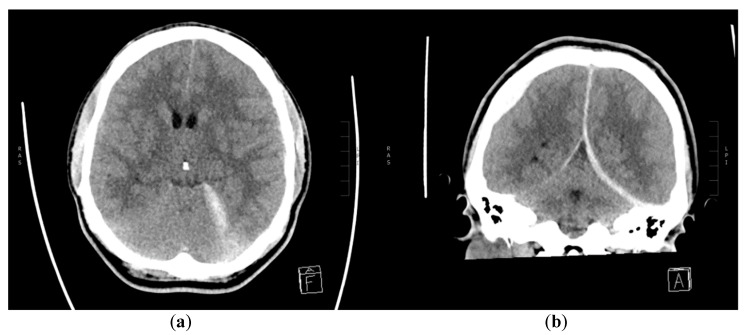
Non-contrast CT scanning of the brain on the day of first admission. (a) Axial and (b) coronal multiplanar reconstruction (MPR) showing left-sided aSDH along tentorium cerebelli and posterior interhemispheric fissure. The right-sided epidural hematoma (EDH) is also seen on image (b).
